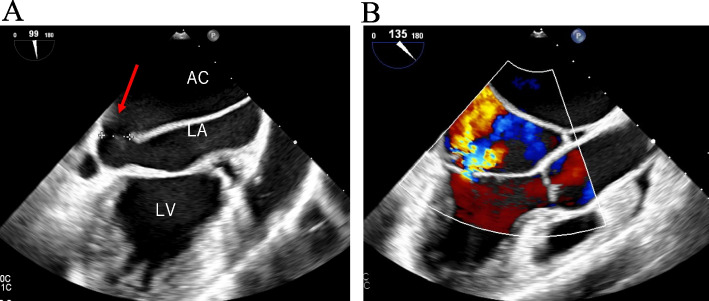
Preoperative transesophageal echocardiography. A The left atrium was divided into the accessory chamber and the main chamber by the anomalous membrane (red arrow). B The diameter of the fenestration was 11 mm with a peak gradient of 20 mmHg (blue arrow). C Moderate mitral regurgitation associated with mitral annual enlargement was found. AC, accessory chamber; MC, main chamber
